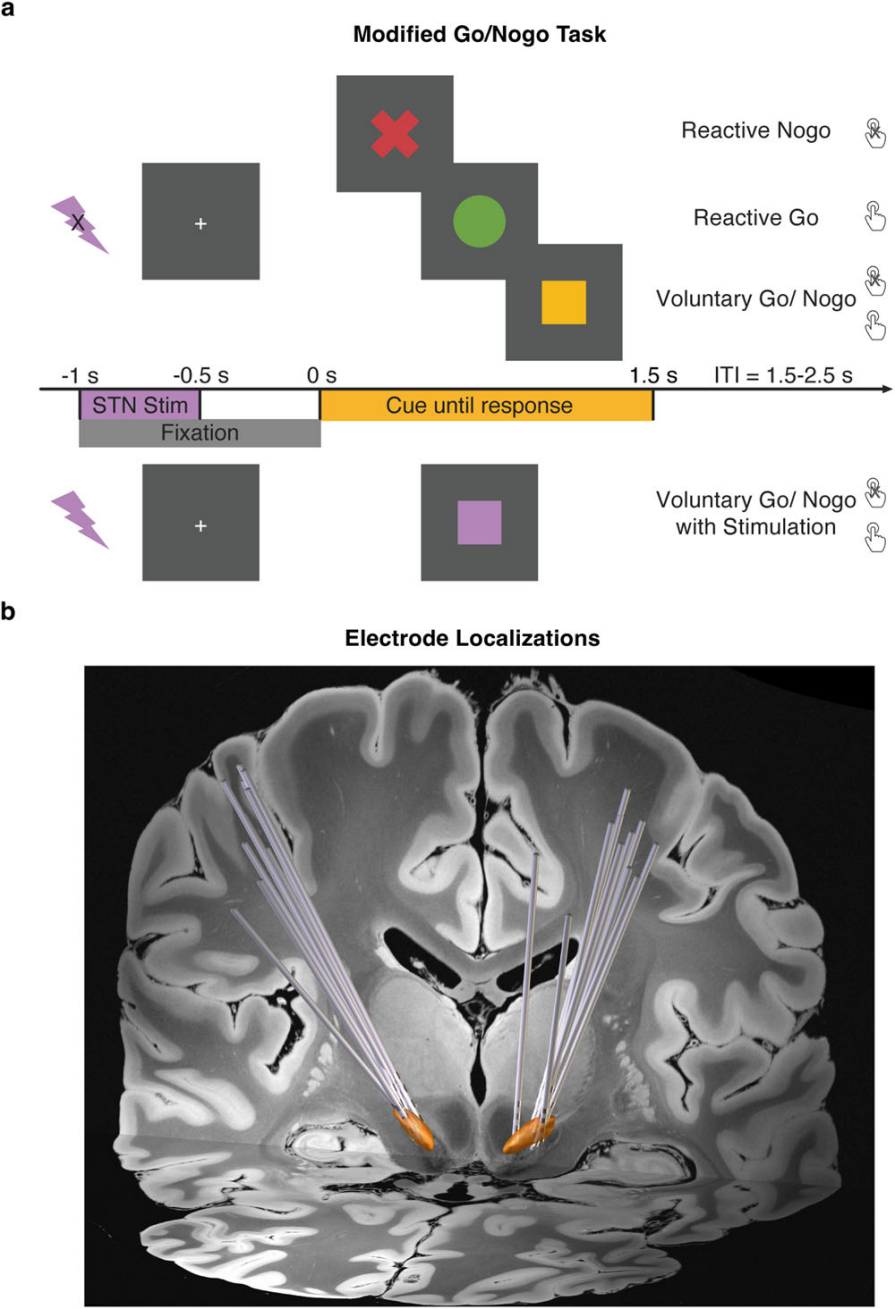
The modified Go/Nogo task paradigm. a The modified Go/Nogo task consisted of ‘reactive’, ‘voluntary’, and ‘voluntary with stimulation’ trials. In the ‘reactive’ trials, patients were instructed to either respond with a key press, or make no response, as indicated by the cue of a red cross or green circle respectively. In the ‘voluntary’ trials, patients made free choices between a key press response, or no response, as indicated by the yellow square cue. In the ‘voluntary with stimulation’ trials, patients made free choices as well. Right subthalamic stimulation at 130 Hz was delivered at 1000 ms prior to the cue onset, and lasted for 500 ms, which was indicated by a purple square. b Electrode reconstruction in Montreal Neurological Institute (MNI) space, with the coronal view displayed on a 7 T T1-weighted structural MRI template. The STN is highlighted in orange.
